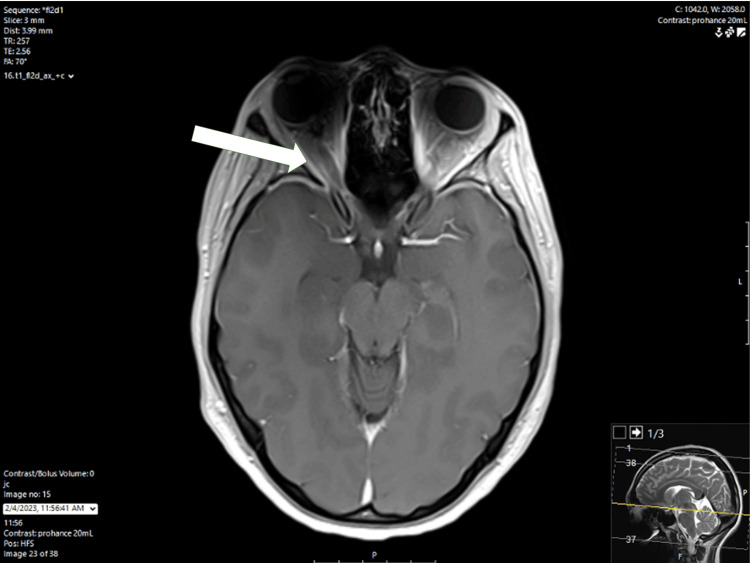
MRI of the brain with/without contrast MRI: magnetic resonance imaging
